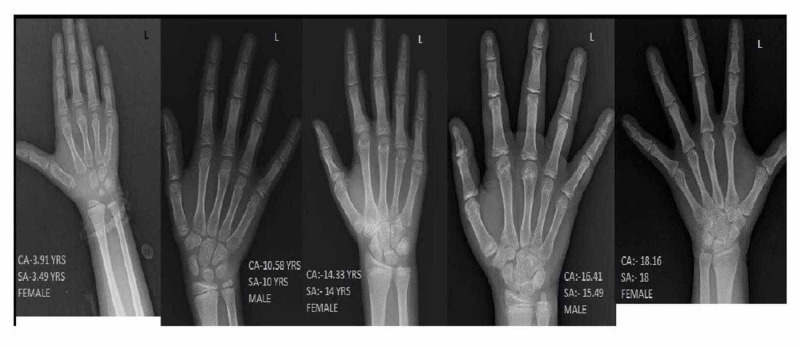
X-ray images of left hand of different chronological and skeletal age in both sex
